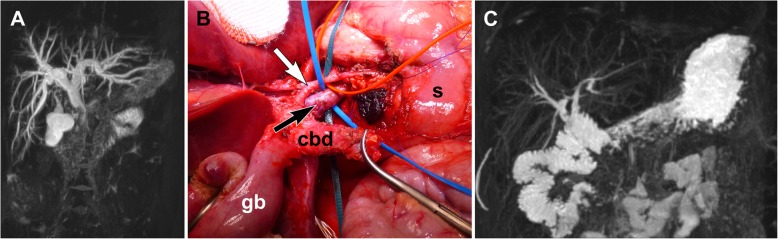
Rhabdomyosarcoma of the biliary tract. a Preoperative MRI image showing the tumor located at the distal region of the common bile duct. b Intraoperative aspect of the tumor region. c Postoperative follow-up MRI image showing the biliodigestive anastomosis and no evidence of local relapse. Abbreviations and symbols in the figure: cbd: common bile duct; gb: gallbladder; s: stomach; white arrow: hepatic artery; dark arrow: portal vein
